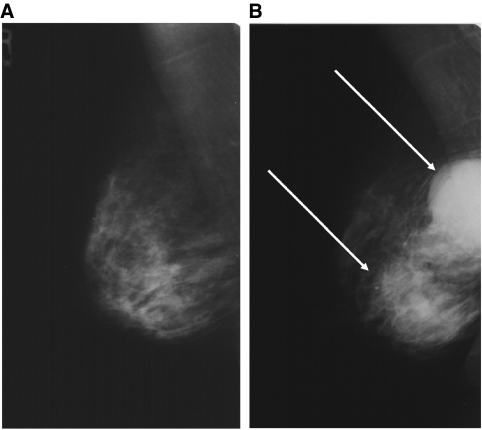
(A) The mammogram of a 58-year old patient referred with an 8-week history of a clinically benign (E2) lump inferior to the right nipple, reported as normal at initial assessment and subsequent review. A breast ultrasound (US) of the palpable lump by a consultant radiologist found no suspicious abnormality and the patient was discharged. (B) Mammogram taken 2 years later when the patient re-presented stating that the lump had enlarged and clinical examination showed a clinically malignant (E5) abnormality, shows an obvious large bilobed mass (arrows) suspicious for malignancy, which was confirmed on US. A core biopsy showed a metaplastic carcinoma.
